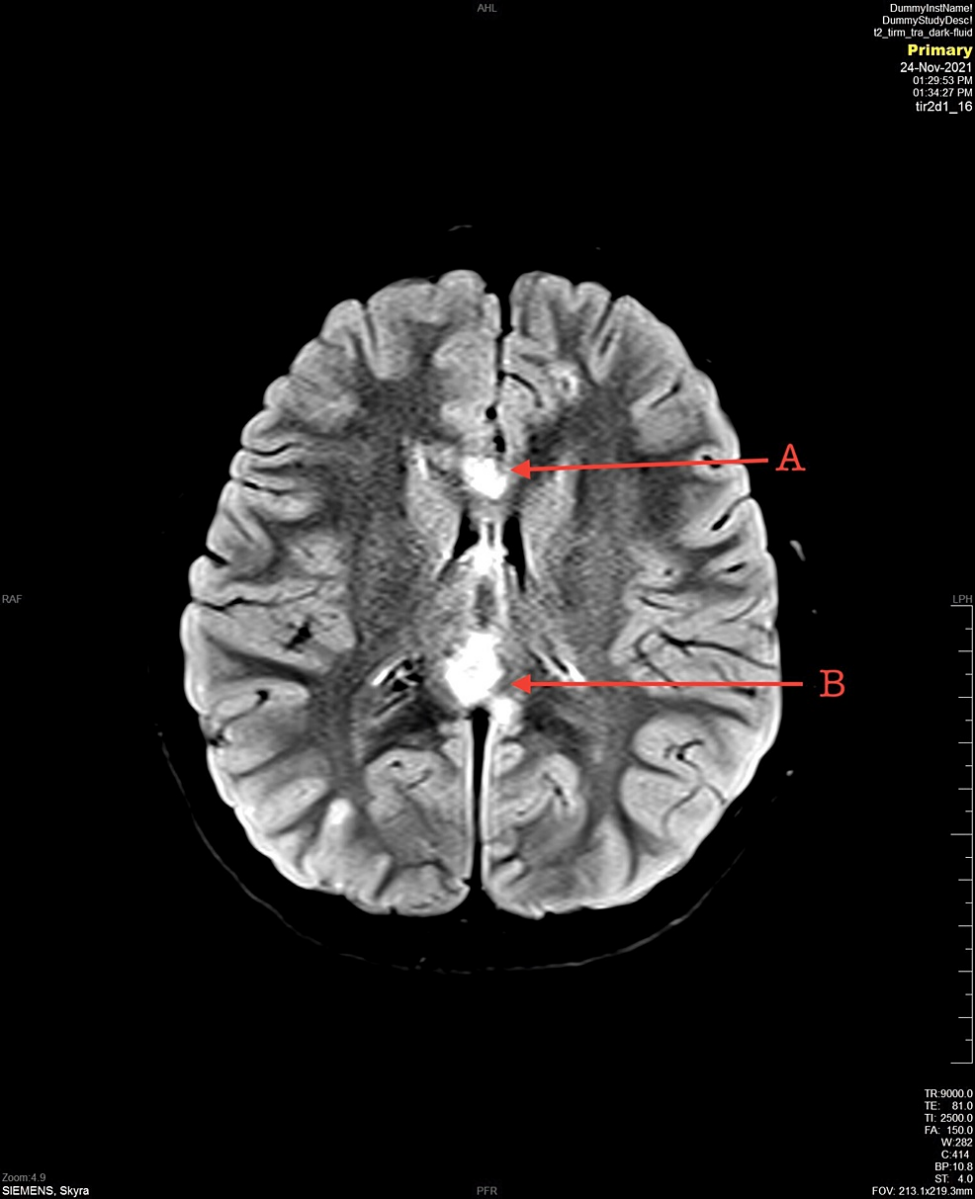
An abnormal fluid attenuated inversion recovery (FLAIR) signal intensity occupying the corpus callosum including genu (A) and splenium parts (B).
